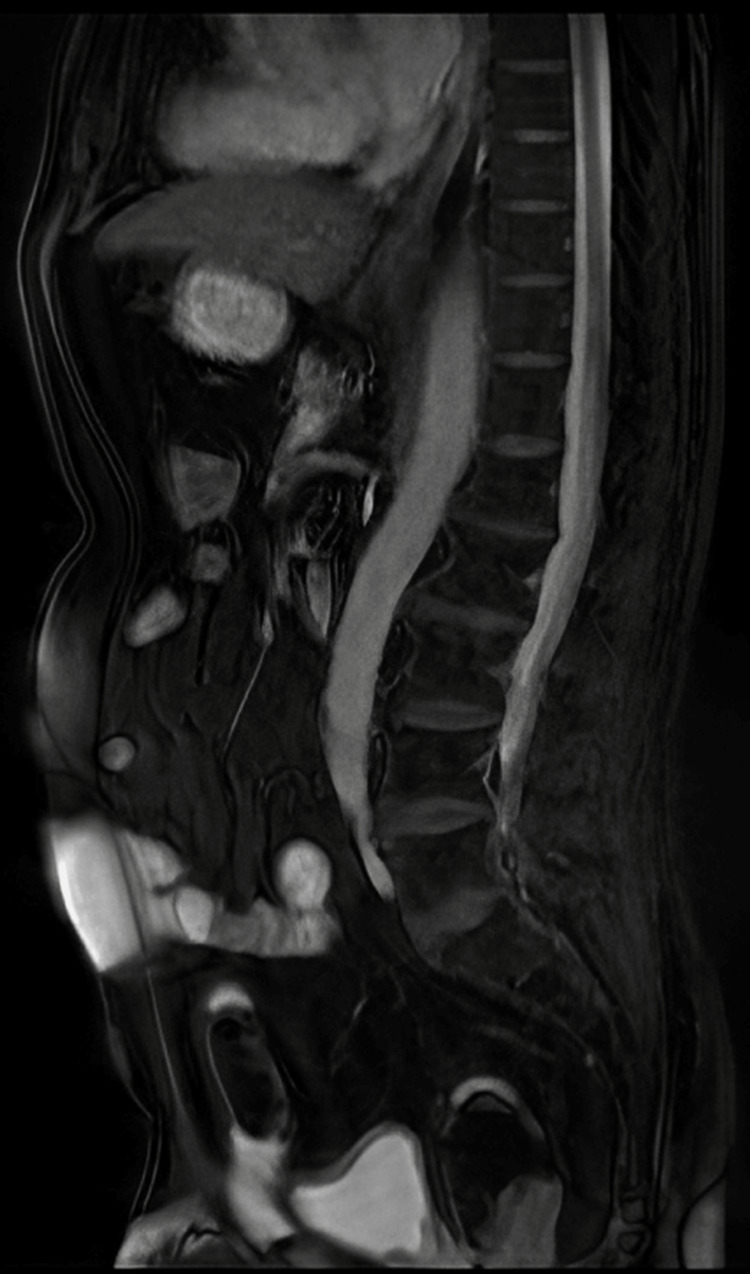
MRI - spine Screening of the spine using MRI T2-weighted images demonstrates no other discrete lesions noted elsewhere MRI: magnetic resonance imaging
